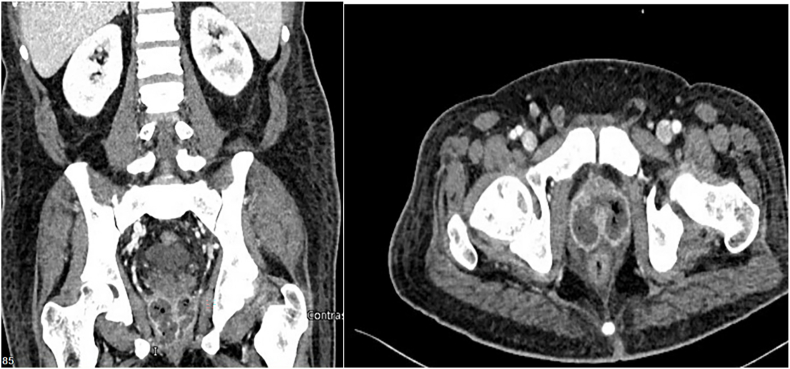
CT abdomen and pelvis with contrast ten days after the 1st resection showed a slight interval reduction in the collection size within the prostatic gland.
